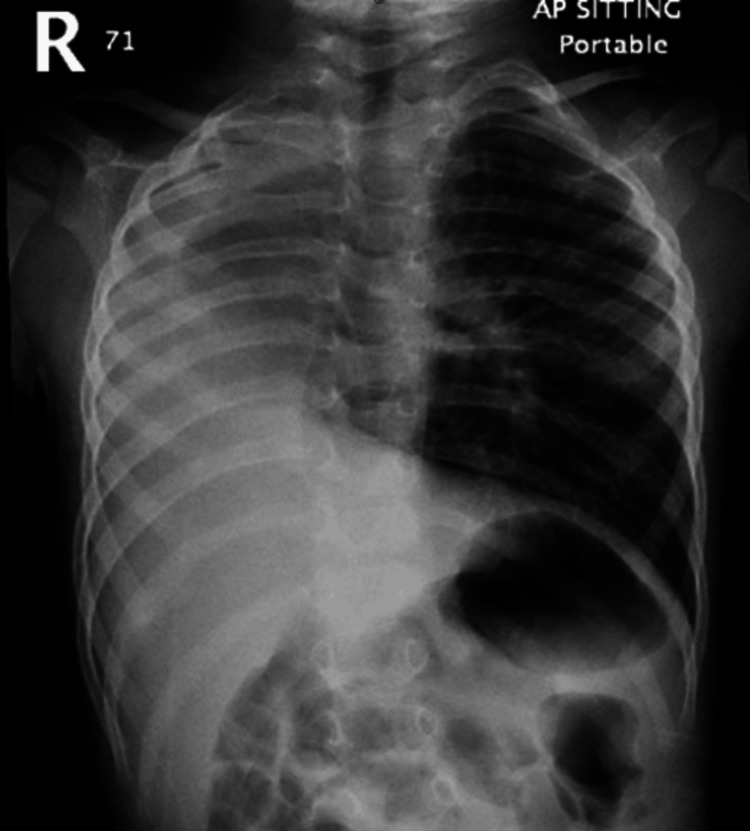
Chest X-ray showing dextrocardia and partial opacification of the right hemithorax and hyperinflation of the left lung
